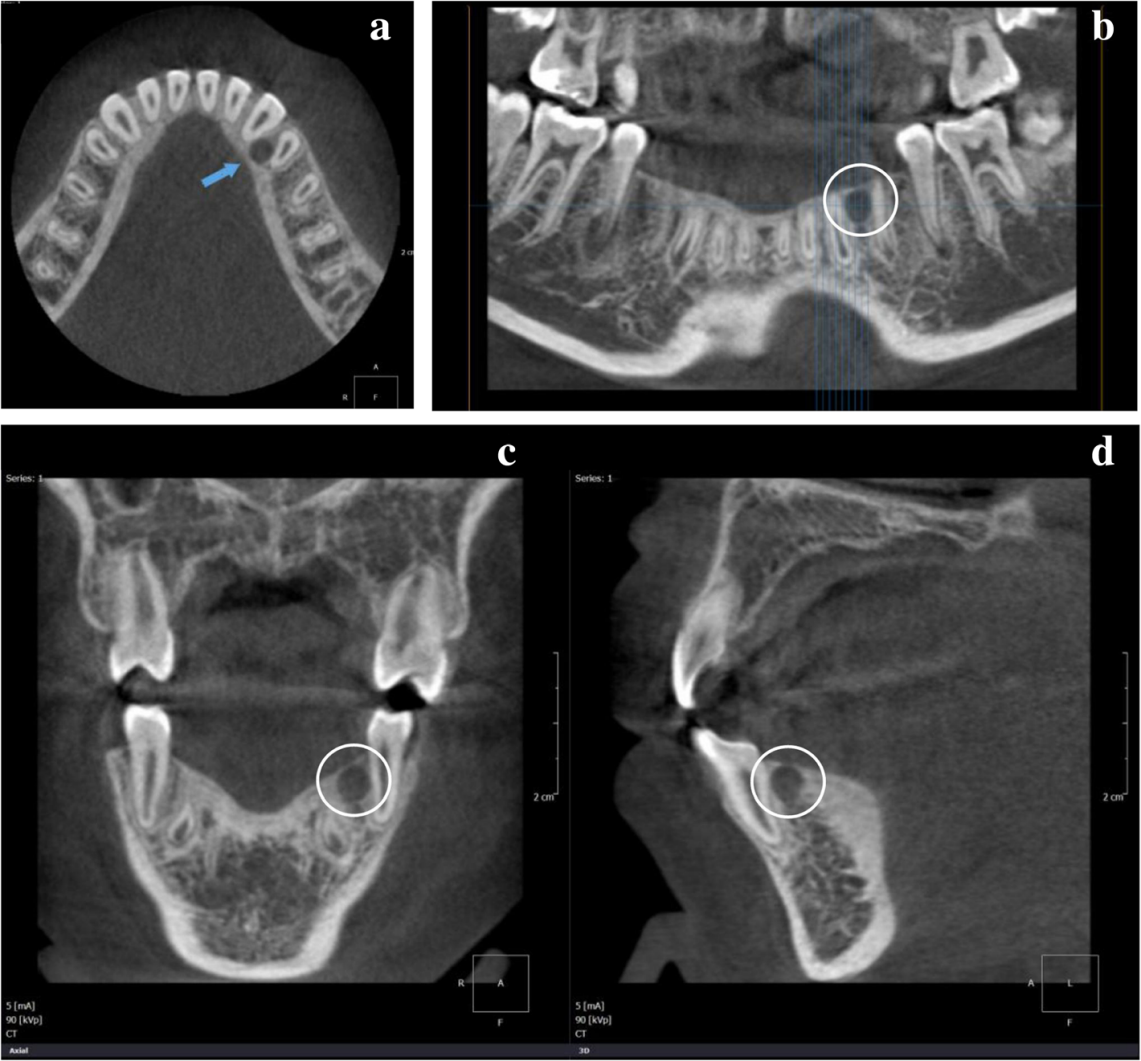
Radiographic findings of POT. a Cone-beam CT showing a round cavity with defined cortical border that is mesiolingual to the root of tooth 34. b-d Panoramic radiograph demonstrates well-defined radiolucency (circled area) in the mandibular left region
